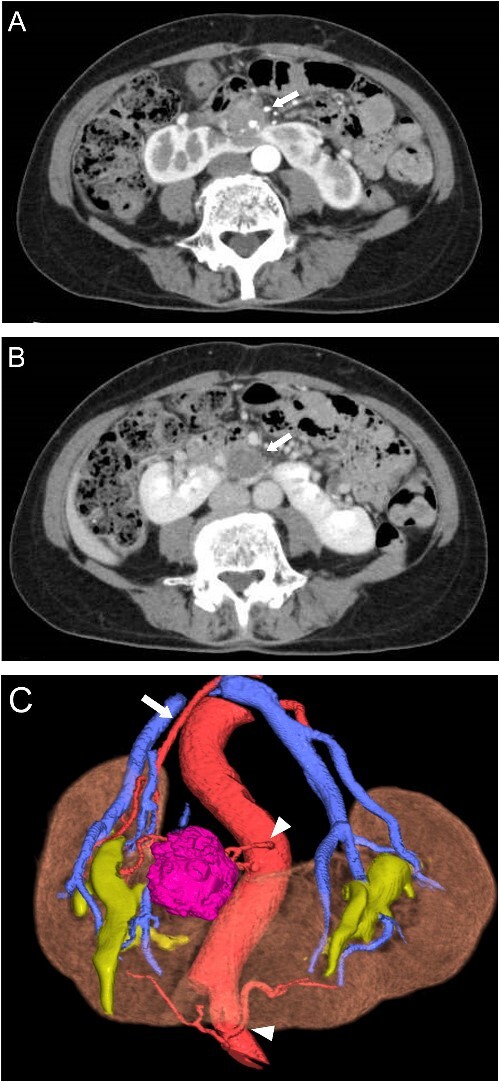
Preoperative CT scans. (A) Contrast-enhanced CT showed heterogeneous enhancement of the tumor in the arterial phases (arrow). (B) The cystic area of the tumor had no enhancement in the venous phase (arrow). (C) 3D-CT demonstrated one artery arising from the aorta to the right kidney (arrow) and two arteries supplying the isthmus and tumor arising from the ventral side of the aorta and left common iliac artery (arrowhead).
